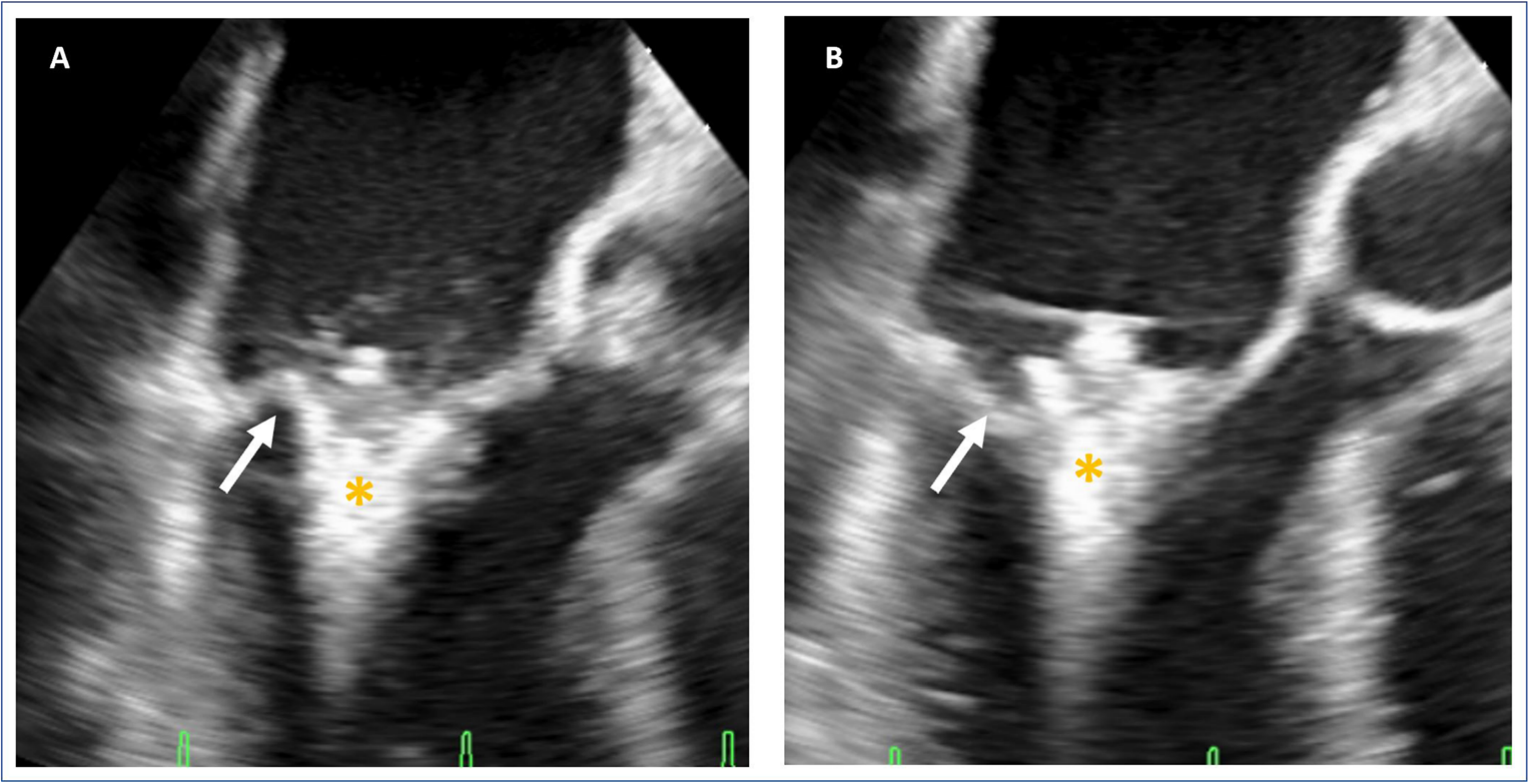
Perforation. Presentation of an intraprocedural leaflet perforation during grasping. A Transesophageal commissural view demonstrating the grasp of the leaflet showing the posterior leaflet (*arrow*) still on the clip (*). B Transesophageal commissural view showing the perforated posterior leaflet (*arrow*). *MitraClip
